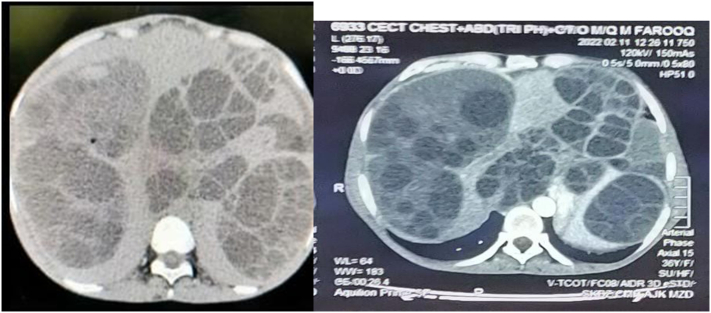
Transverse section of CT scan.
